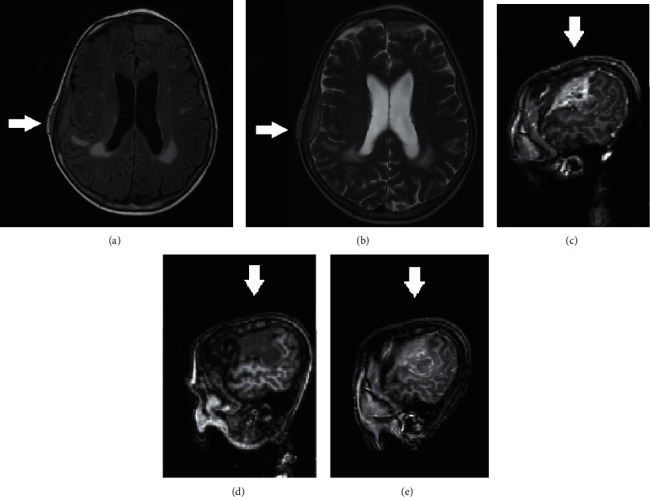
Cranial MR showing a solid epidural 2 × 2.8 × 5 cm mass, originating from the bone, in the right parietal region with (a) homogeneously low signal intensity on T1-weighted images and (b) homogeneously high signal intensity on T2 and FLAIR images. (c–e) A 6.2 × 3.2 × 1.3 cm mass, infiltrating the bone, with similar structure and contrast enhancement is seen in mainly the left frontal area.
